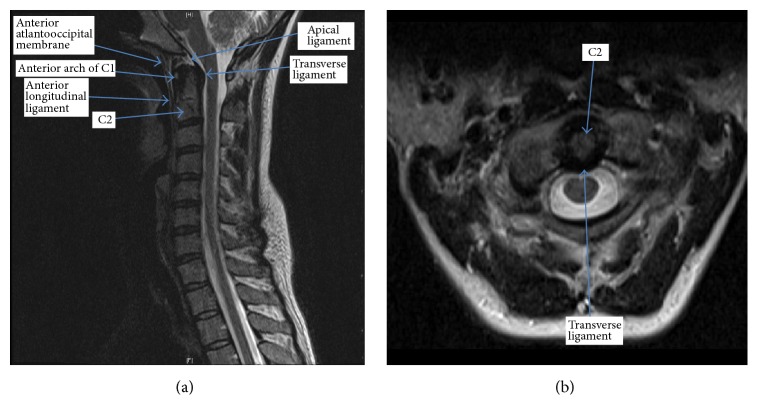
Sagittal (a) and axial (b) MRI images depicting the ligaments of the upper cervical spine.
